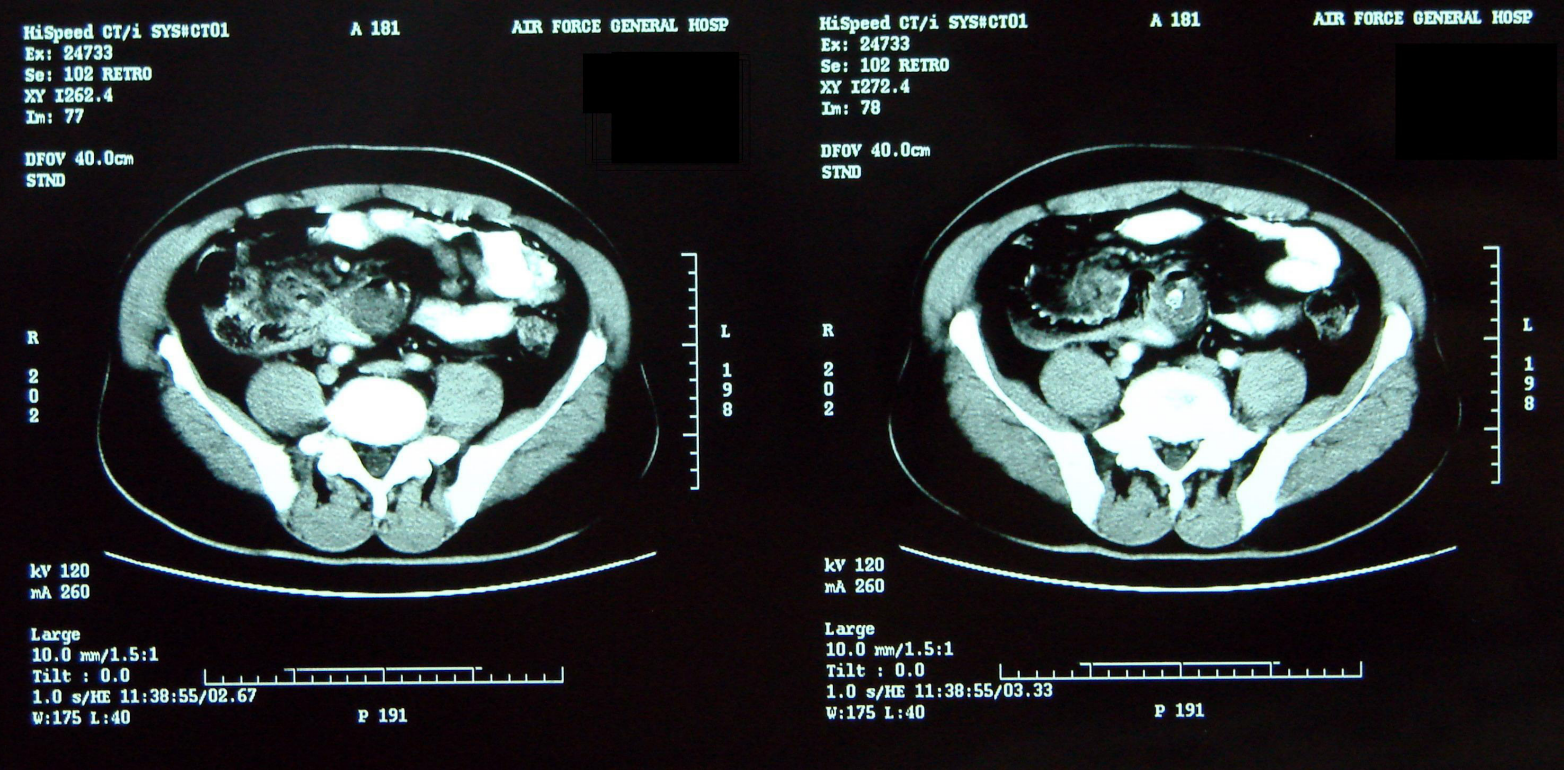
Abdominal CT scan. Sclerosing mesenteritis in a 52-year-old male with a long history of abdominal pain. Axial CT scan demonstrates a solid soft-tissue mass with calcification in the right lower abdomen, which was related to small bowel and mesentery. The mass had a typical "fat ring sign" and a calcified core.
